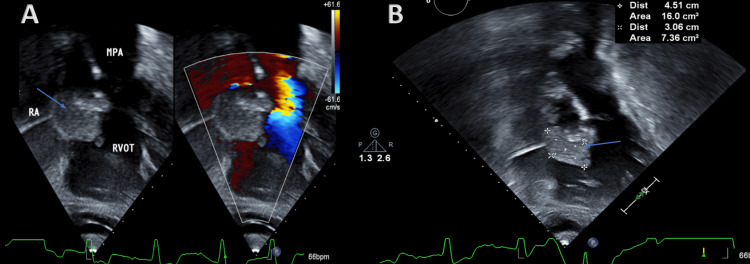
Transthoracic echocardiography (A) Subcostal view showing a hyperechoic mass (blue arrow) protruding from the right atrium (RA) to the right ventricle (RV). Color Doppler imaging was negative for flow within the mass. (B) The mass measurement in two dimensions was 4.51 x 3.06 cm.
